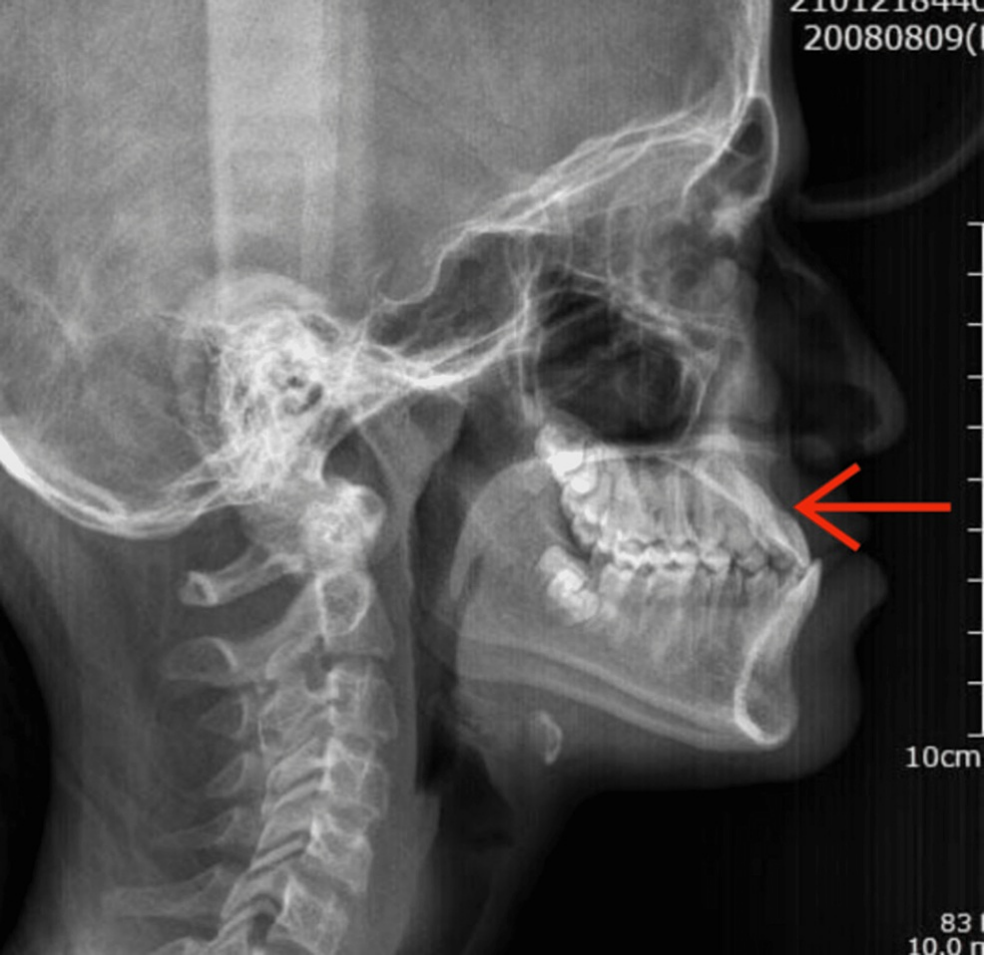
Preoperative radiograph. Depicts the preoperative radiograph showing Class III malocclusion. The arrow mark depicts the retrognathic maxilla in the lateral cephalogram.
